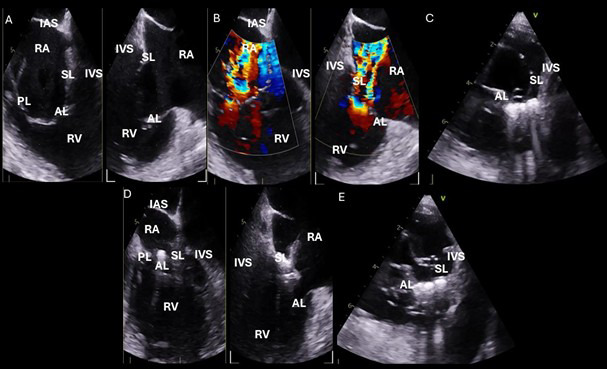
Intraprocedural transesophageal echocardiographic (TEE) views
